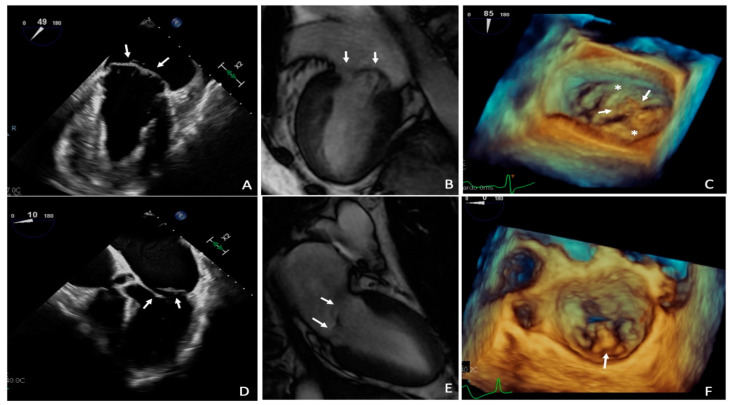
Multimodality assessment of MVP due to Barlow’s disease and due to fibroelastic deficiency; 2D TEE (A) and CMR (B) images show thickening and prolapse of both mitral valve leaflets in Barlow’s disease; 3D TEE (C) confirms mitral prolapse involving multiple segments of both leaflets (asterisks). Two ruptured chordae (arrows) are also visible; 2D TEE (D) and CMR (E) show thin mitral valve leaflets (arrows) with posterior leaflet flail confirmed by 3DTEE ((F), arrow) in fibroelastic deficiency. CMR: Cardiac Magnetic Resonance; FED: Fibroelastic deficiency; TEE: Transeoesophageal echocardiography.
